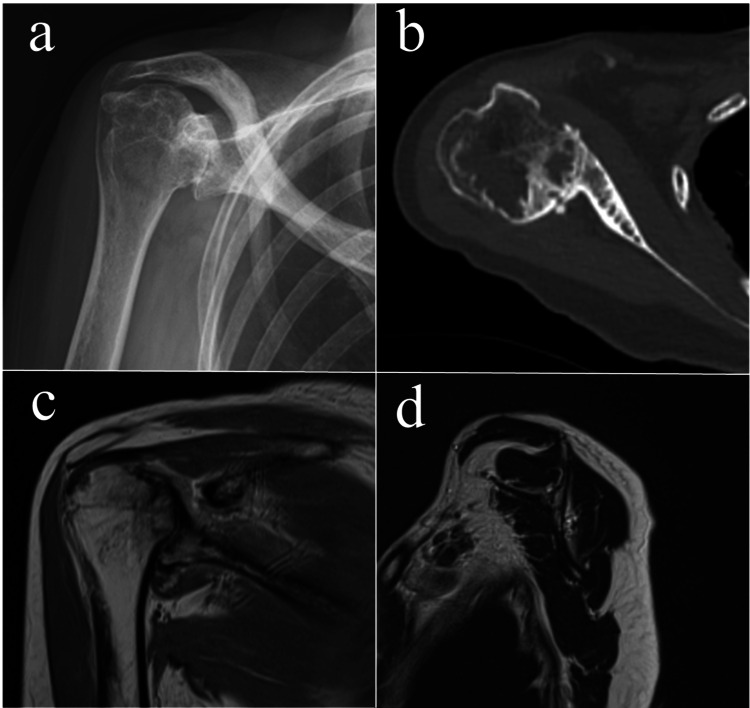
Preoperative imaging findings. (a) Plain radiograph (anteroposterior view). (b) Computed tomography (axial view). (c) Magnetic resonance imaging (T2-weighted image, coronal view). (d) Magnetic resonance imaging (T2-weighted image, sagittal view).
